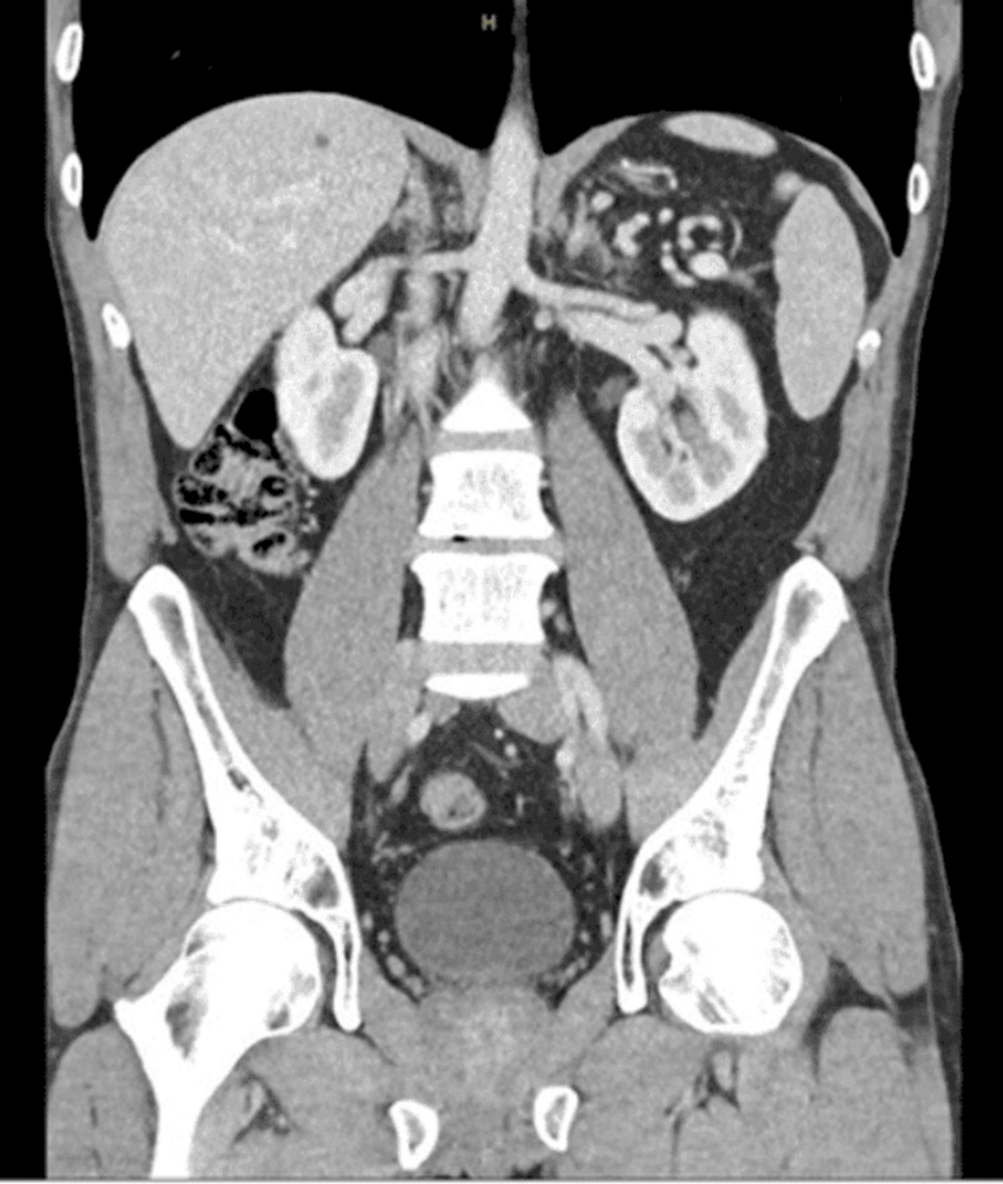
CT of the abdomen and pelvis (coronal view) Contrast-enhanced abdominopelvic CT demonstrates normal findings.
